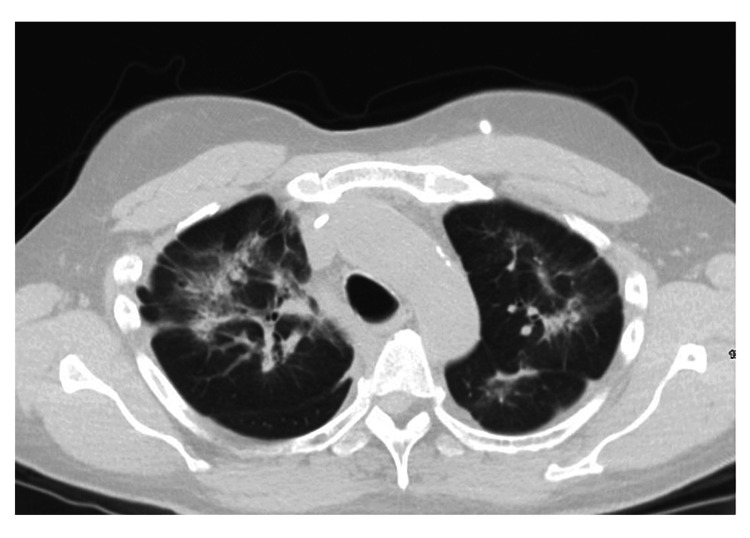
CT of the chest demonstrating bilateral interstitial opacities
